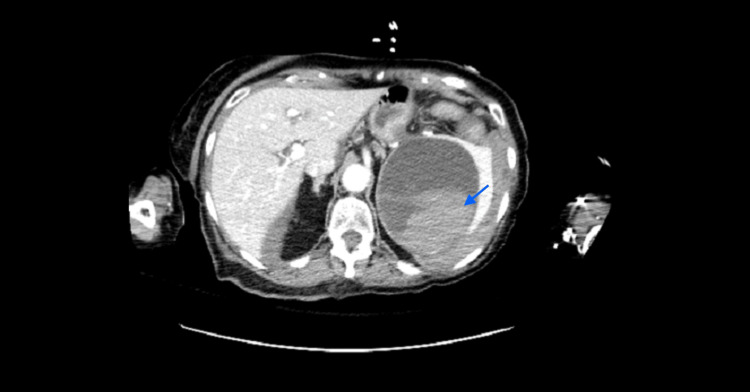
Axial abdominal CT imaging showing the spleen with a patchy splenic hyperdensity and dense perisplenic fluid. Splenic lesion identified by a blue arrow. CT, computed tomography
